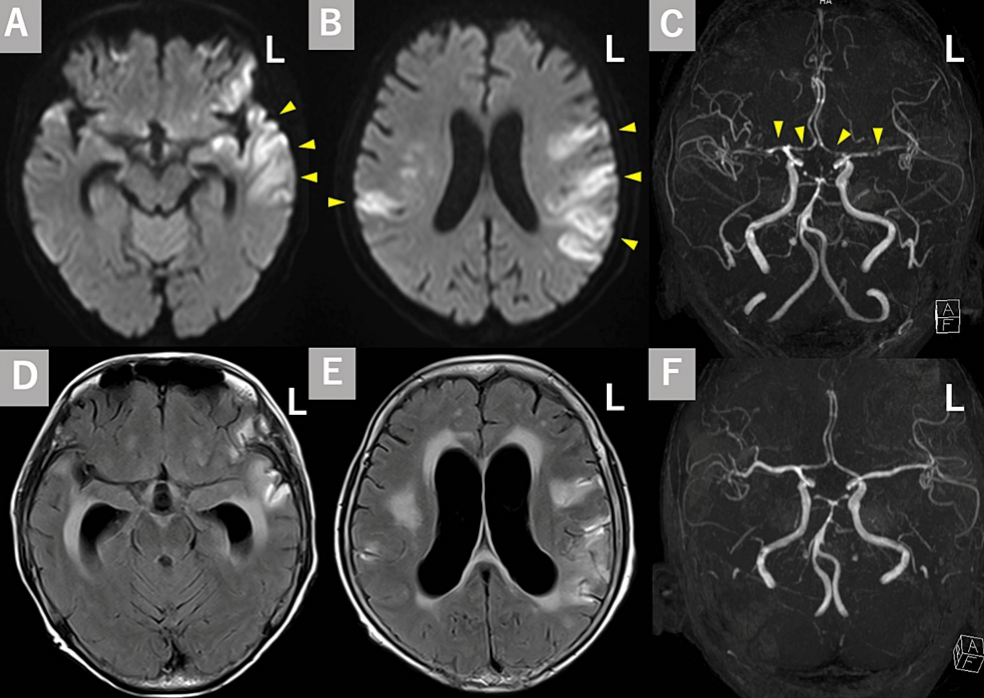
Imaging findings of the present case A, B: Axial diffusion-weighted imaging (DWI) showed hyperintense lesions in the bilateral middle cerebral artery territory (arrowheads). C: Magnetic resonance angiography (MRA) showed multiple stenoses in the bilateral middle cerebral artery, anterior cerebral artery, and basilar artery (arrowheads). D, E: Fluid attenuated inversion recovery (FLAIR) in follow-up showed hydrocephalus. F: Follow-up MRA showed an improvement in arterial stenosis.
